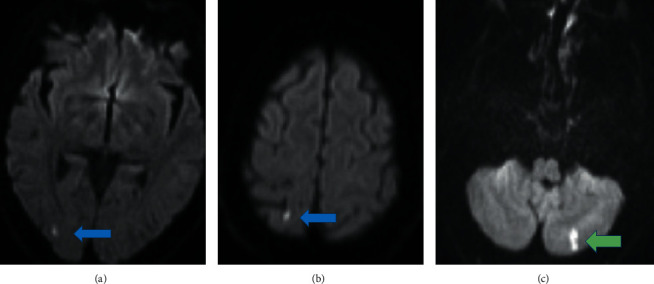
MRI of brain without contrast. Blue arrows: occipital and parietal tiny infarcts. Green arrow: cerebellar infarct.
